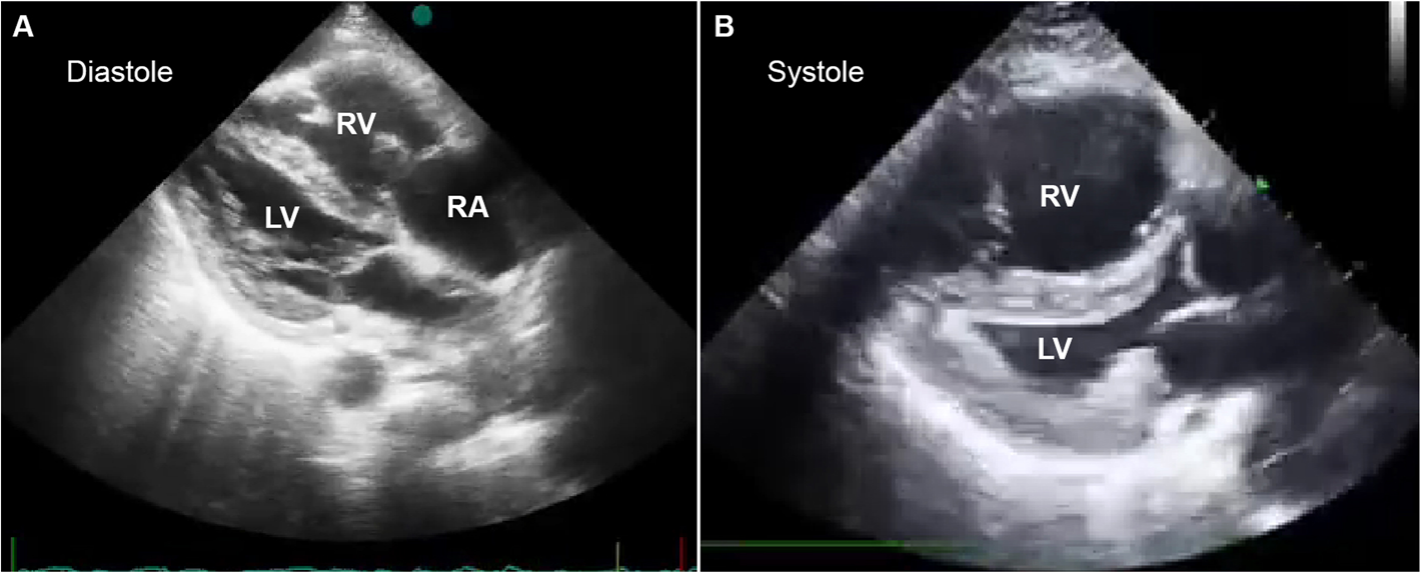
Bedside echocardiography shows poor contractility of the dilated right ventricles during diastole a and systole b
